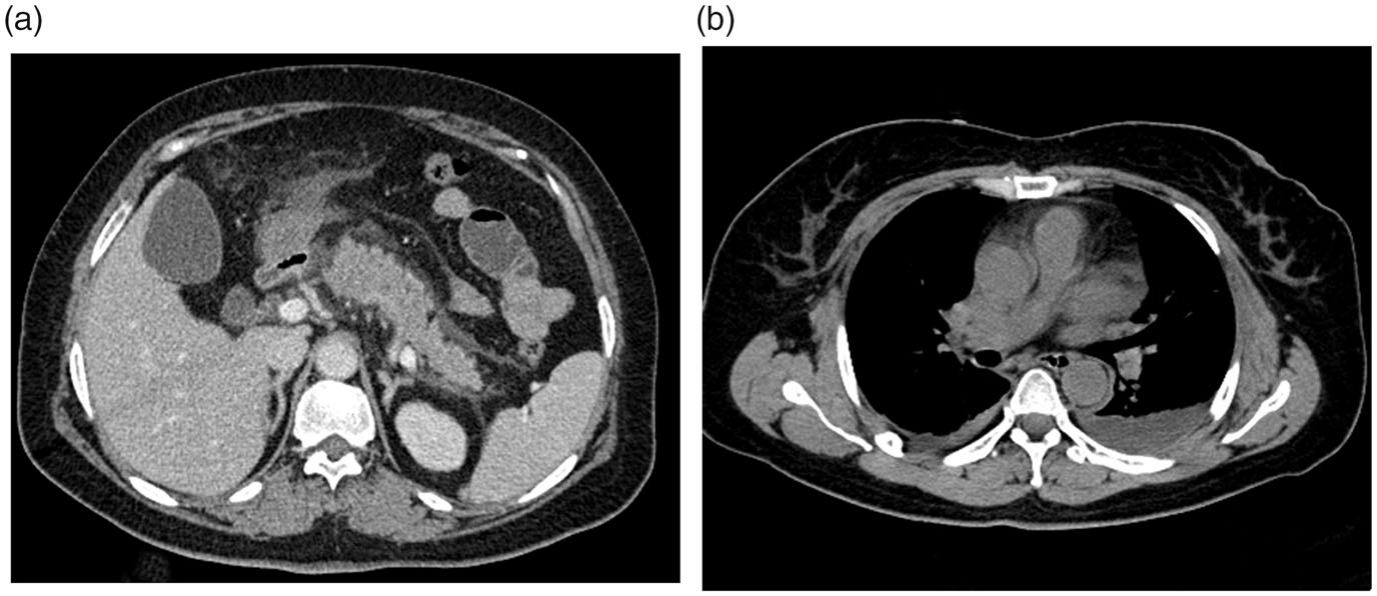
Mild interstitial oedematous pancreatitis (IEP) in a 57-year-old female (CRP level of 28 mg/L, Ranson, BISAP, Marshall, APACHE II, CTSI, and EPIC of 1, 1, 0, 4, 4, and 3 points, respectively; there was no infection, procedure, ICU admission, organ failure, or death on this patient). Abdominal axial contrast-enhanced CT at the portal phase (A) showed there was a small amount of acute peripancreatic fluid collection (APFC) around the pancreas. Axial chest image CT (B) showed there was a small amount of bilateral pleural effusion.
